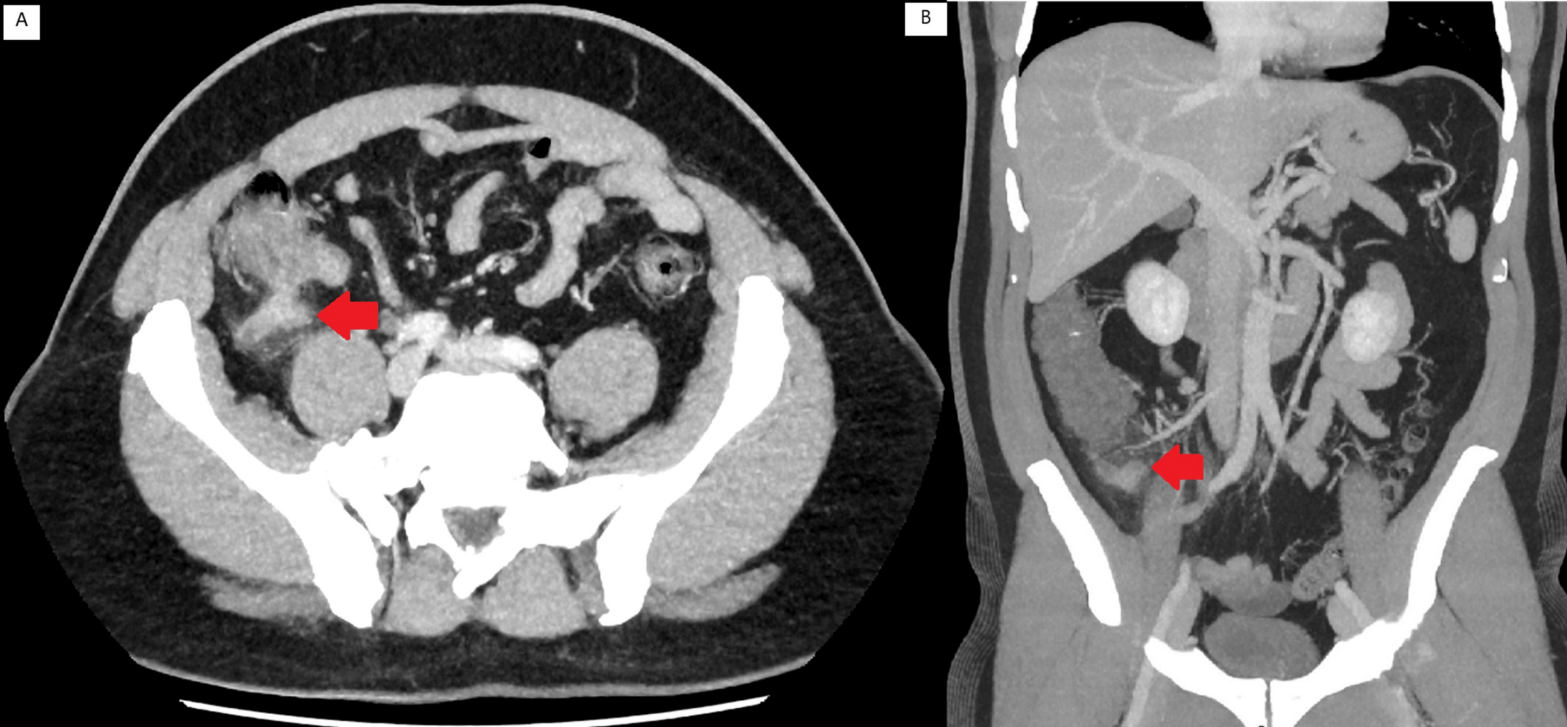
CT images of appendicitis with appendiceal diverticula The above are images taken from the pre-operative CT scan. Appendiceal diverticula are noted and indicated by the red arrows. Given the inflammatory fat stranding and clear margins, this was initially perceived to be a contained perforation and or abscess, which had formed from the appendix.
